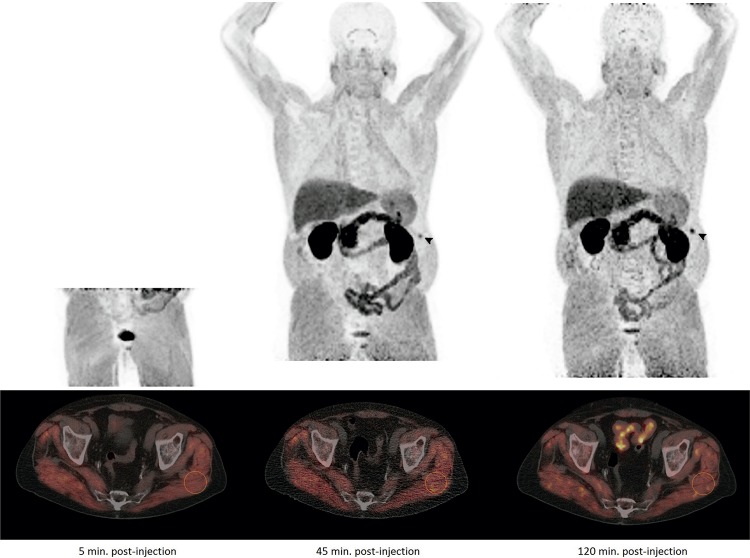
MIP and fused PET/CT images acquired at 5 min, 45 min and 120 min after intravenous injection of 18F-Choline. An intense and difuse uptake of radiotracer was observed in all the series of the study. The muscle activity was measured by circular ROI in gluteus maximus muscle (axial fused images) demonstrating stable activity throughout the exploration. Incidentally, a fracture of the eleventh left rib arch was also observed (head-arrow).
